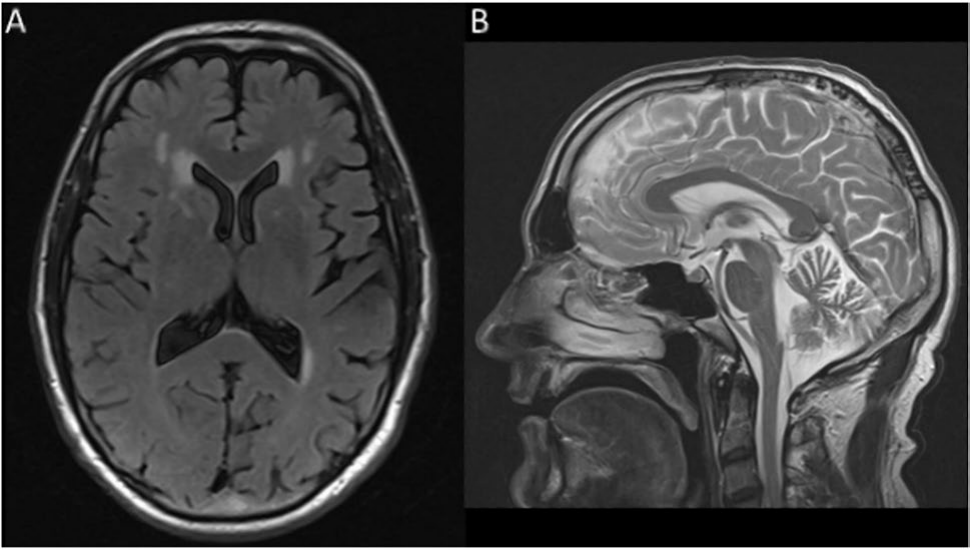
MRI scan of the E146G proband at 9 months following symptom onset. A Axial FLAIR imaging demonstrates white matter hyperintensities in excess for age focussed on the frontal lobes. B Sagittal T2-weighted imaging demonstrates atrophy of the cerebellum
